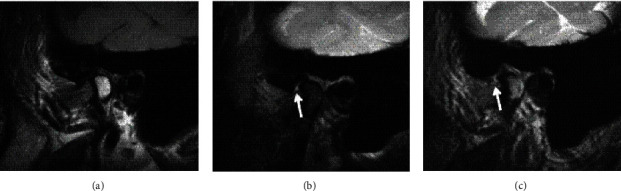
Sagittal MR images of another patient (a) FSE T2-W showing no effusion whereas (b) FS T2W and (c) 3D FIESTA-C show a mild effusion in the inferior joint compartment (arrows).
